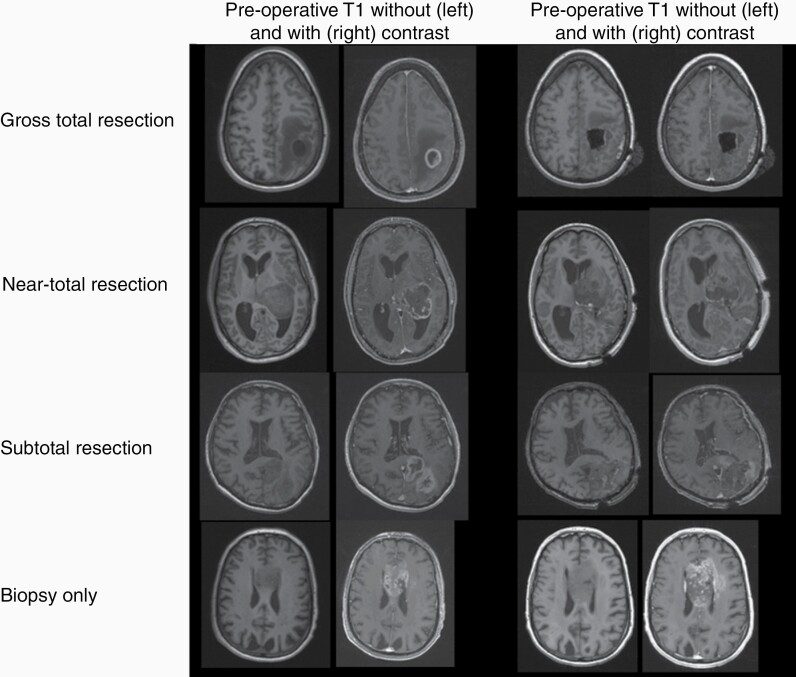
Extent of resection as depicted by T1-weighted sequences without and with gadolinium contrast on preoperative and postoperative magnetic resonance imaging.
